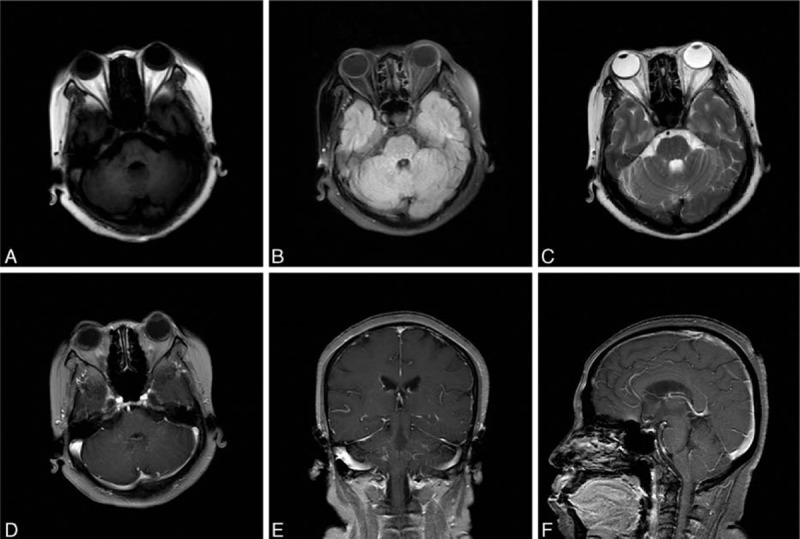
MRI shows resolution of lesions after 4-month immunosuppressive therapy on T1WI (A), T2WI (C), and FLAIR images (B). Axial (D), coronal (E), and sagittal (F) gadolinium-enhanced T1WI show decreased enhancement in the brainstem and the cerebellum. MRI = magnetic resonance imaging.
